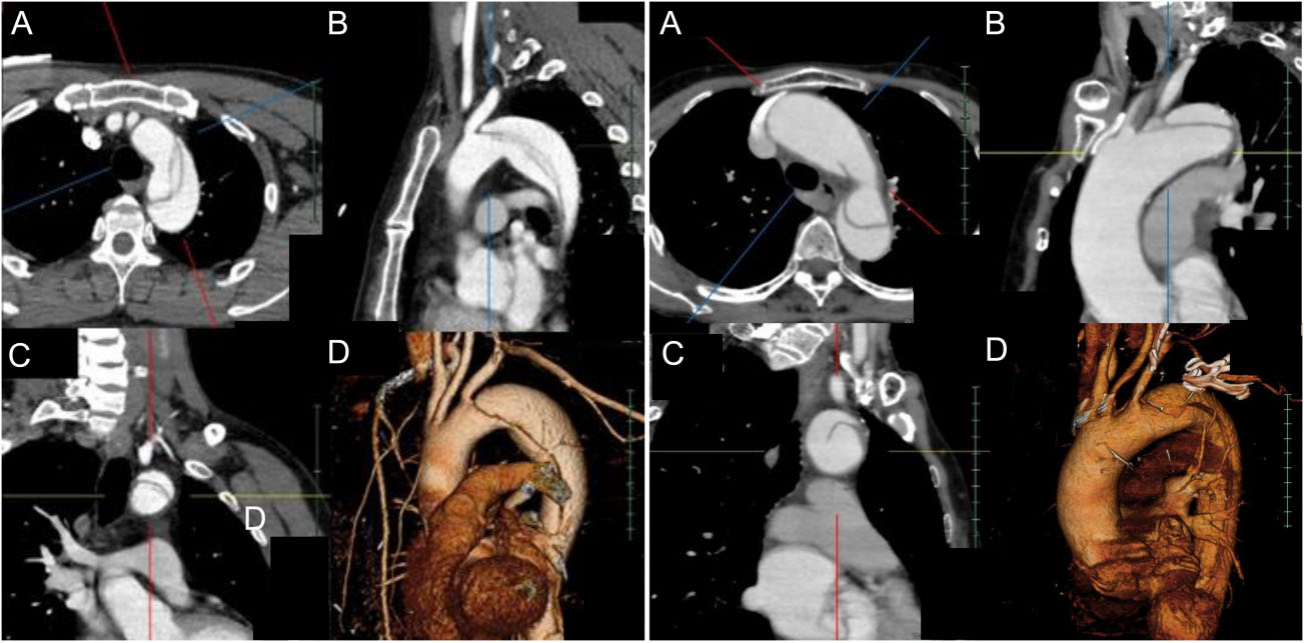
Multiplanar (A–C) and three-dimensional (D) reconstruction computed tomography angiography images obtained in a case of acute type B aortic dissection (left) and a case of acute non-A non-B arch-entry aortic dissection (right).
